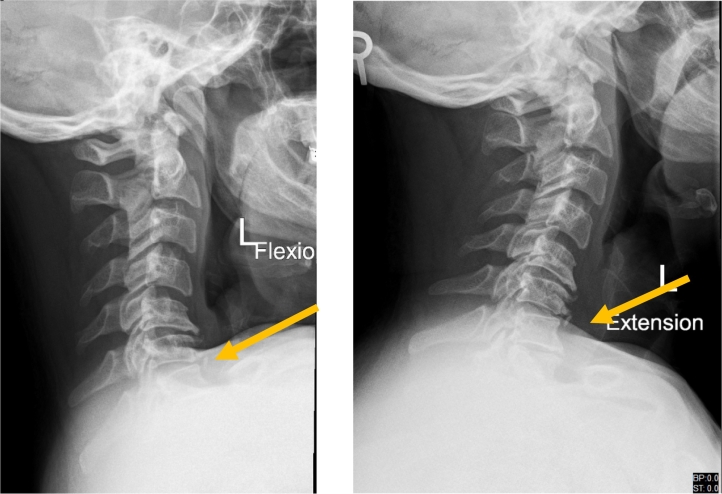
X-ray cervical spine dynamic lateral view showing stable cervical vertebral spine.
